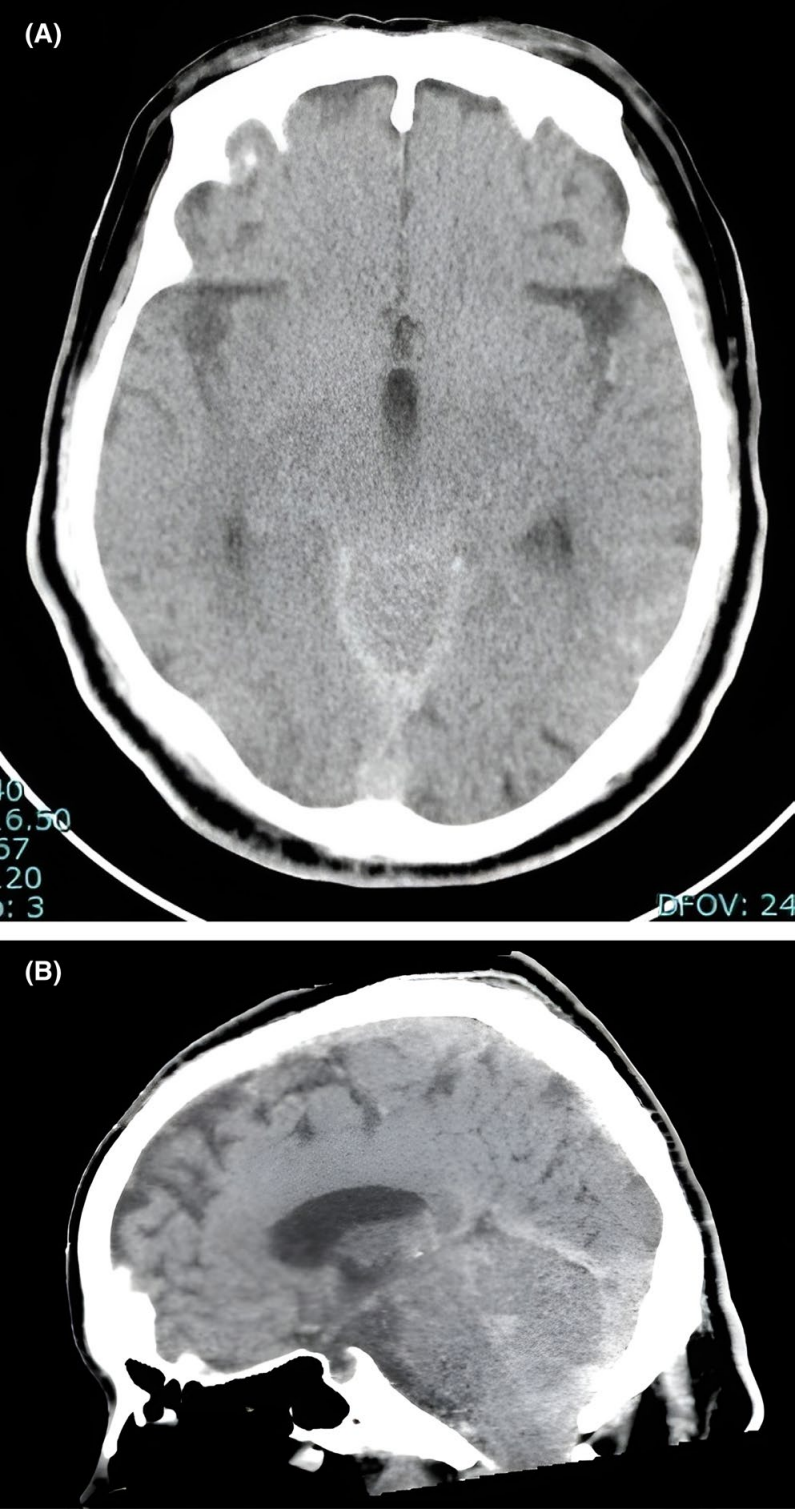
Head CT findings from postmortem imaging. (A) Axial image showing mild subarachnoid hemorrhage and intraventricular hemorrhage. The red arrow indicates mild subarachnoid and intraventricular hemorrhage on axial imaging. (B) Sagittal image showing no evidence of cerebral herniation or brain injury that could have caused cardiopulmonary arrest. The red arrow indicates mild subarachnoid and intraventricular hemorrhage on sagittal imaging.
